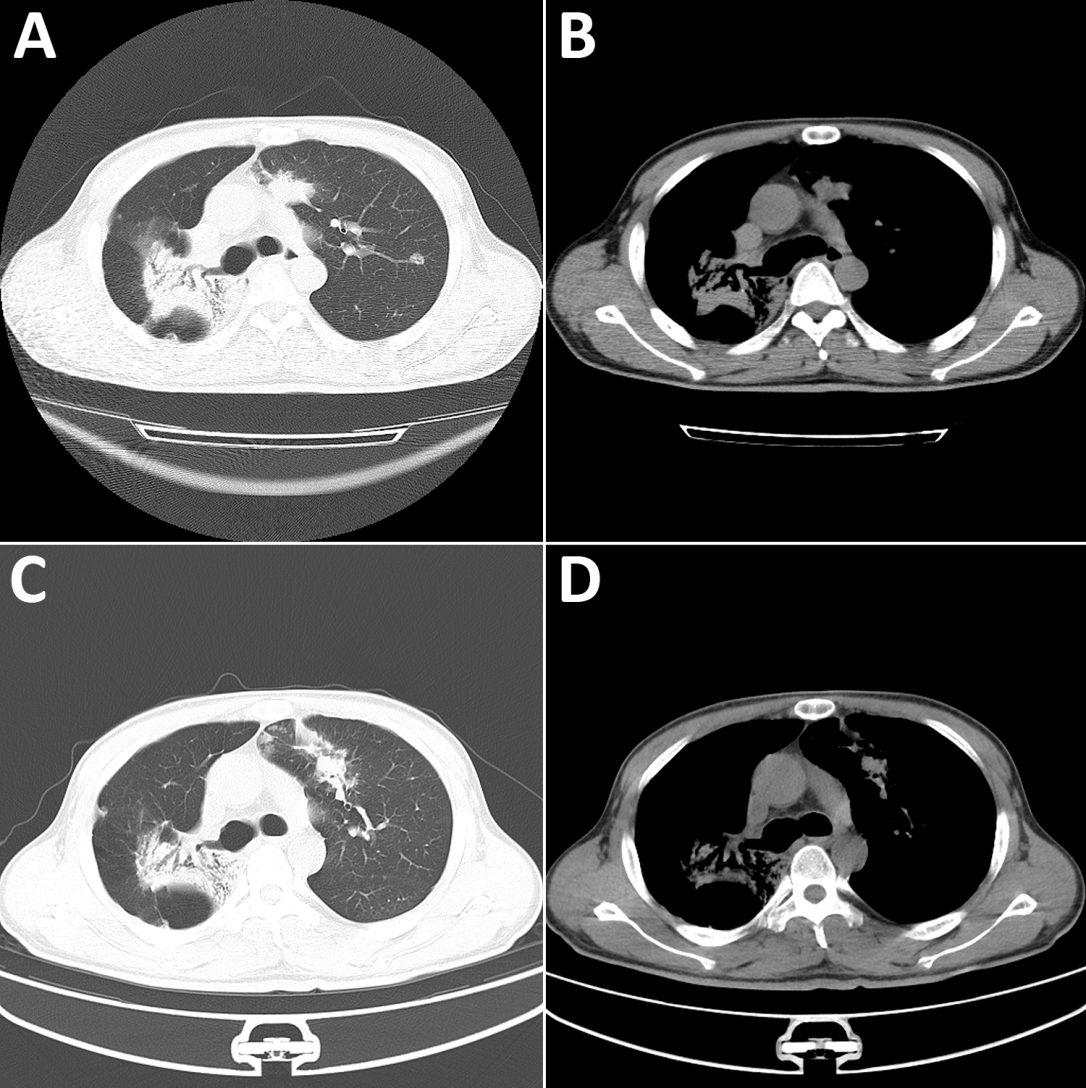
Chest computed tomography (CT) images from a patient with disseminated blastomycosis mimicking tuberculosis, China. A, B) Chest CT at first admission revealed bilateral lobar infiltrates (A) and patchy opacities (B). C, D) Chest CT taken 2 months later showed resolution of left lung lesions but progression of right lung lesions.
